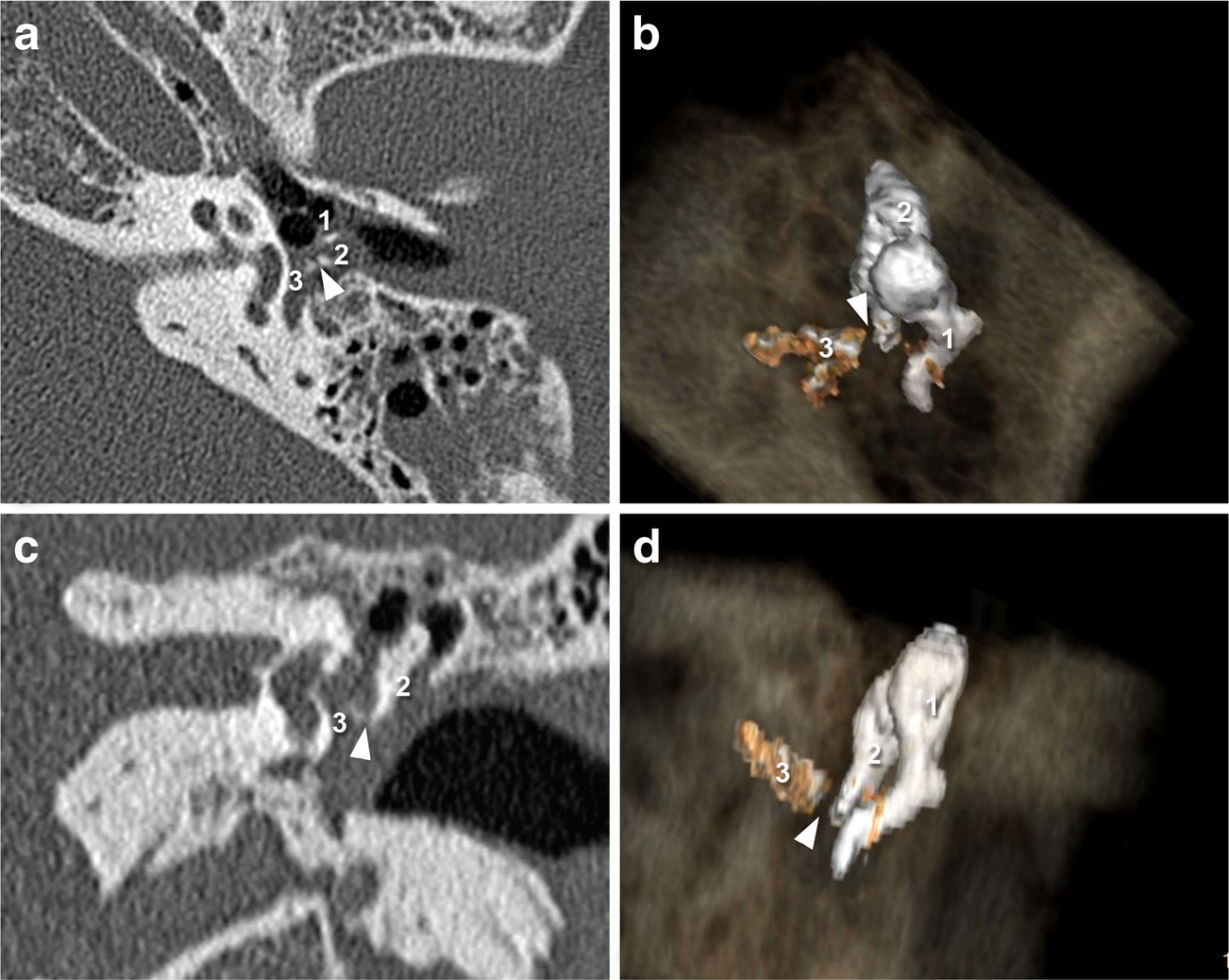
2-D CT images in the axial and oblique planes (a and c) showing a post-traumatic incudo-stapedial joint luxation. The malleus is annotated (1) The oblique plane shows disruption of the ossicular « V », comprising the stapes (3) and the long process of the incus (2). The 3-D CT images (b and d) also reveal the “gap” (*white arrowhead*) between the stapes (3) and the incus (2)
